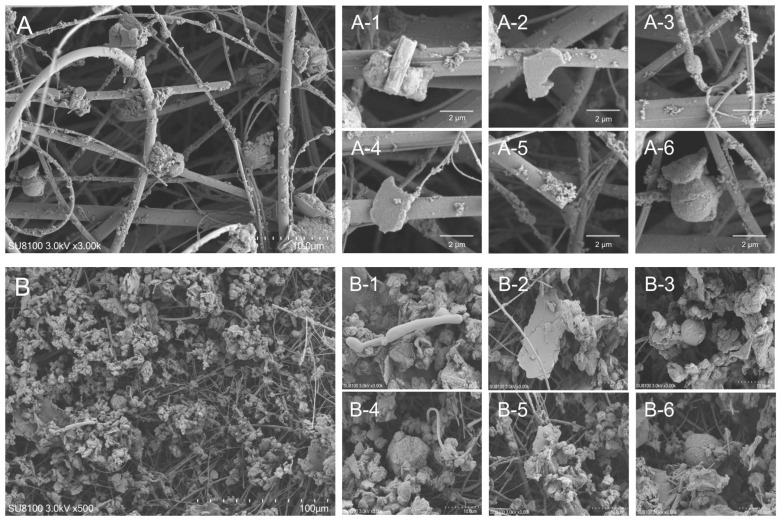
Typical ultrafine structure of PM_2.5_ and PM_10_ samples from a lactating sow barn. (A) PM_2.5_ samples, scale bar = 10 μm; (A-1–A-6) SEM image from X-ray analysis of a single particle of PM_2.5_, scale bar = 2 μm. (B) PM_10_ samples, scale bar = 100 μm; (B-1–B-6) SEM image from X-ray analysis of a single particle of PM_10_, scale bar = 10 μm.
